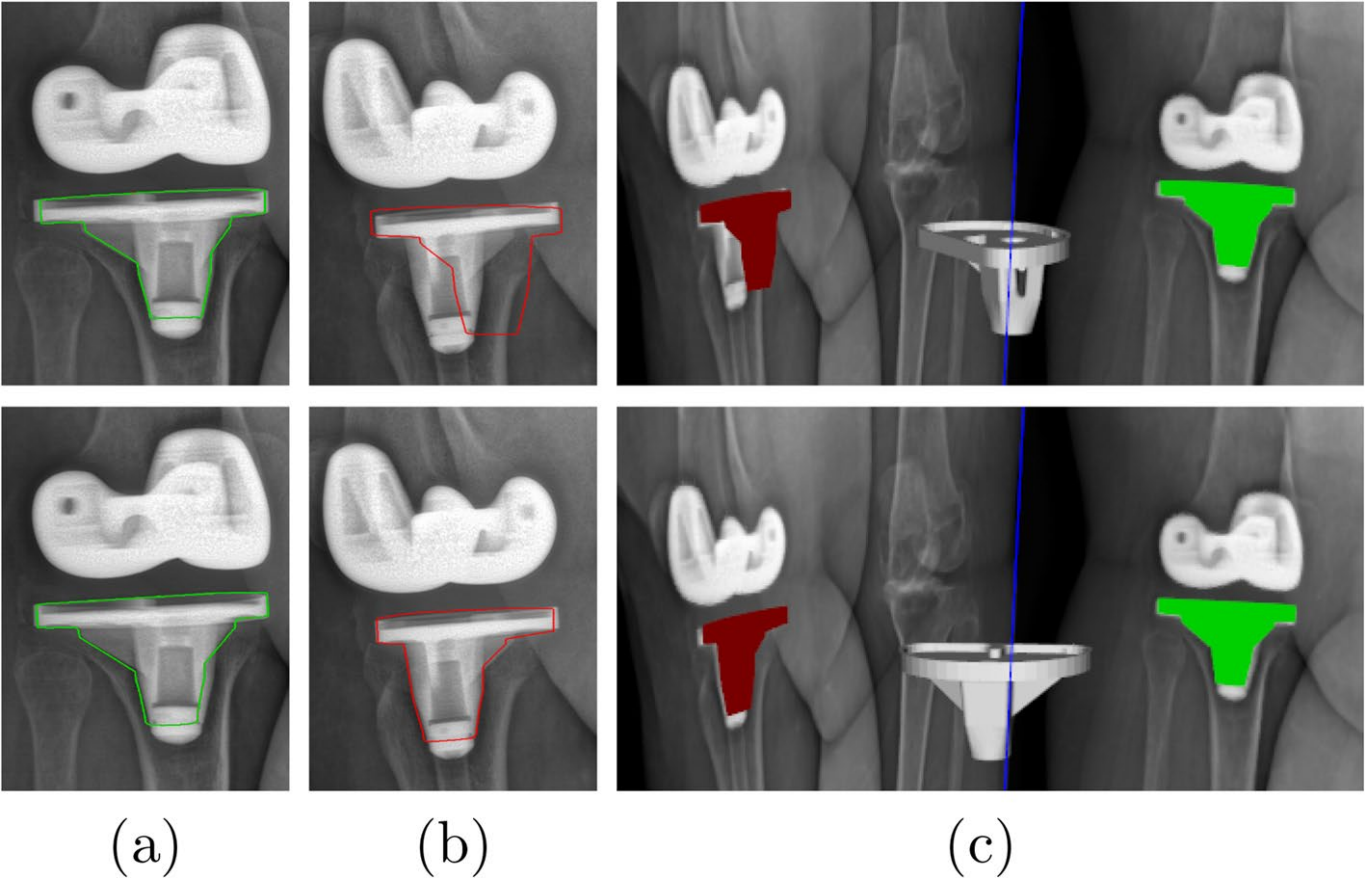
Example of tibial component projection on two X-ray images. From top to bottom : symmetrical and true pose. a, b component external contours projected on 135-degree image and 45-degree image, and (c) 3D view of component projections
